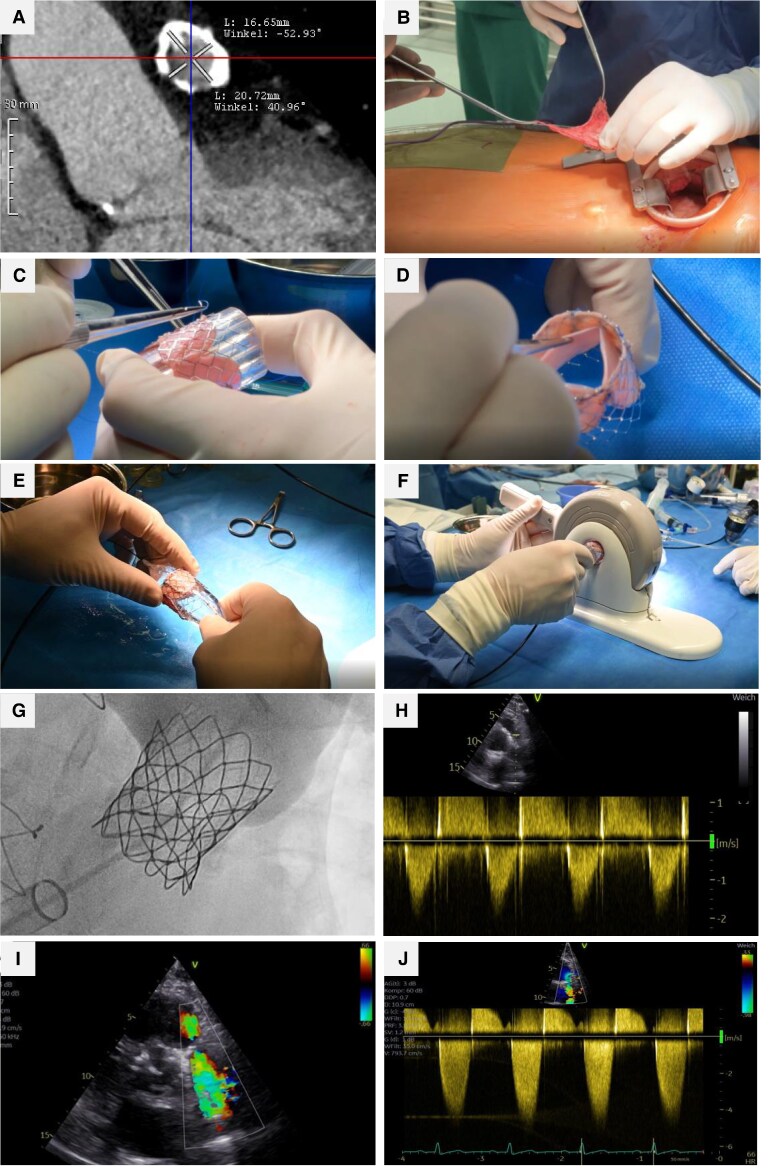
Visualization of GrOwnValve procedure: (*A*) CT-reconstruction and annulus measurement; (*B*) harvesting of pericardium via mini-thoracotomy; (*C*) molding and suturing onto stent frame; (*D*) check for commissure alignment; (*E–F*) crimping and loading onto balloon catheter; (*G*) transcatheter implantation; (*H–J*) echocardiographic assessment after (*H*) and before (*I*, *J*) implantation.
